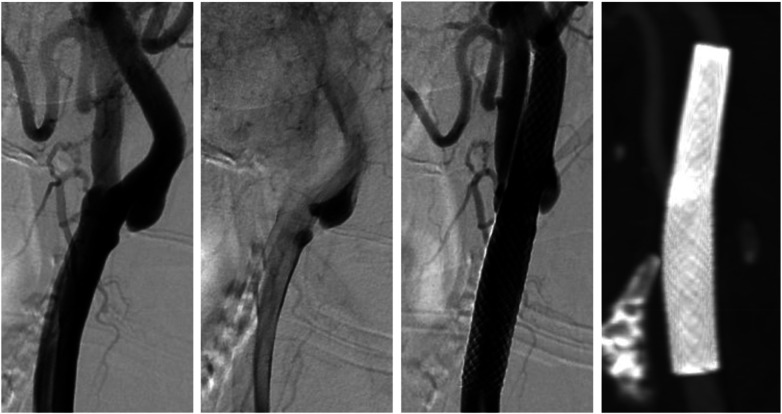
Several weeks after the ischaemic stroke, the patient underwent left carotid artery stenting. (A) Angiographic visualisation of the carotid web. (B) Contrast stagnation confirming haemodynamic disturbance caused by the web. (C) The stent flattens and flattens the web against the vessel wall, isolating the pouch from the bloodstream at the carotid bulb. In the following weeks, endothelialisation of the stent occurs, leading to complete exclusion and thrombosis of the pouch. (D) CT angiography at three-month follow-up demonstrates stent patency and complete thrombosis of the pouch.
